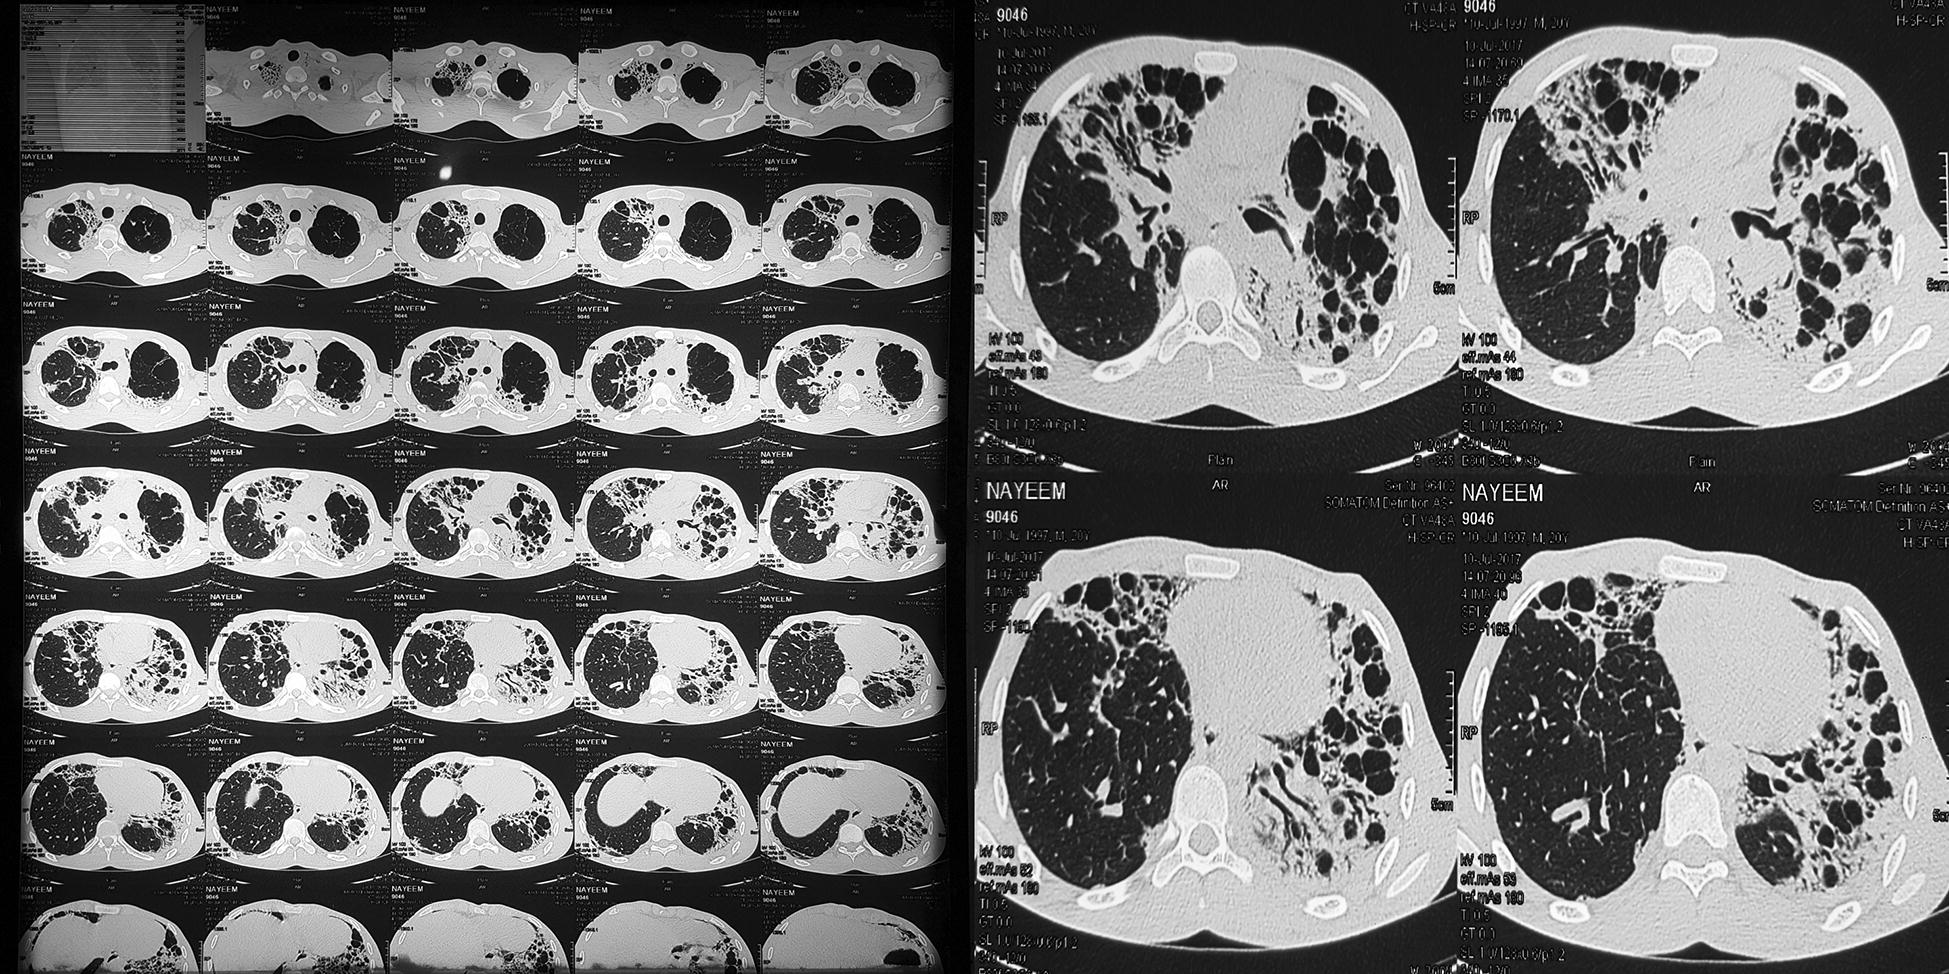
High resolution CT scan of chest demonstrating bilateral pulmonary fibrosis 30 days after Paraquat poisoning
